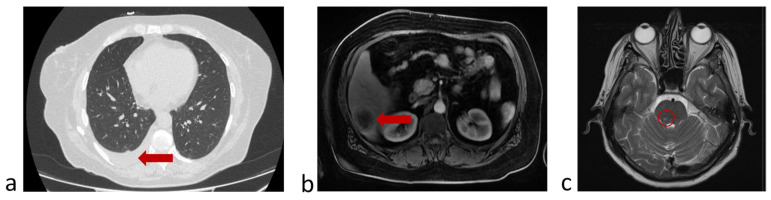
Protocol code 13: (a) abdomen MRI from 20 June 2022: the liver structure is heterogeneous due to several (*n* = 3) focal lesions of an oval shape with clear contours, the largest dimensions being 4.1 × 3.1 cm in S6 subcapsular (indicated by arrow); (b) chest CT from 26 June 2022 revealed right-sided small hydrothorax (indicated by arrow); (c) brain MRI from 24 June 2022: in the substance of the brains on the right, a formation measuring 2 × 5 mm with minimal accumulation of contrast is determined (marked with a circle). All examinations were made before the start of treatment of metastatic cancer.
